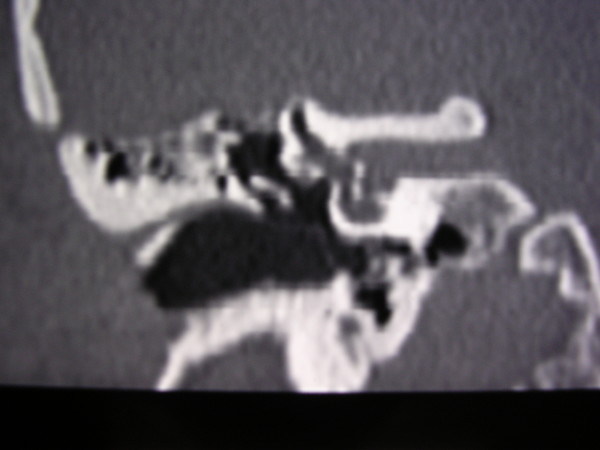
Postoperative temporal bone coronal computed tomography images.
